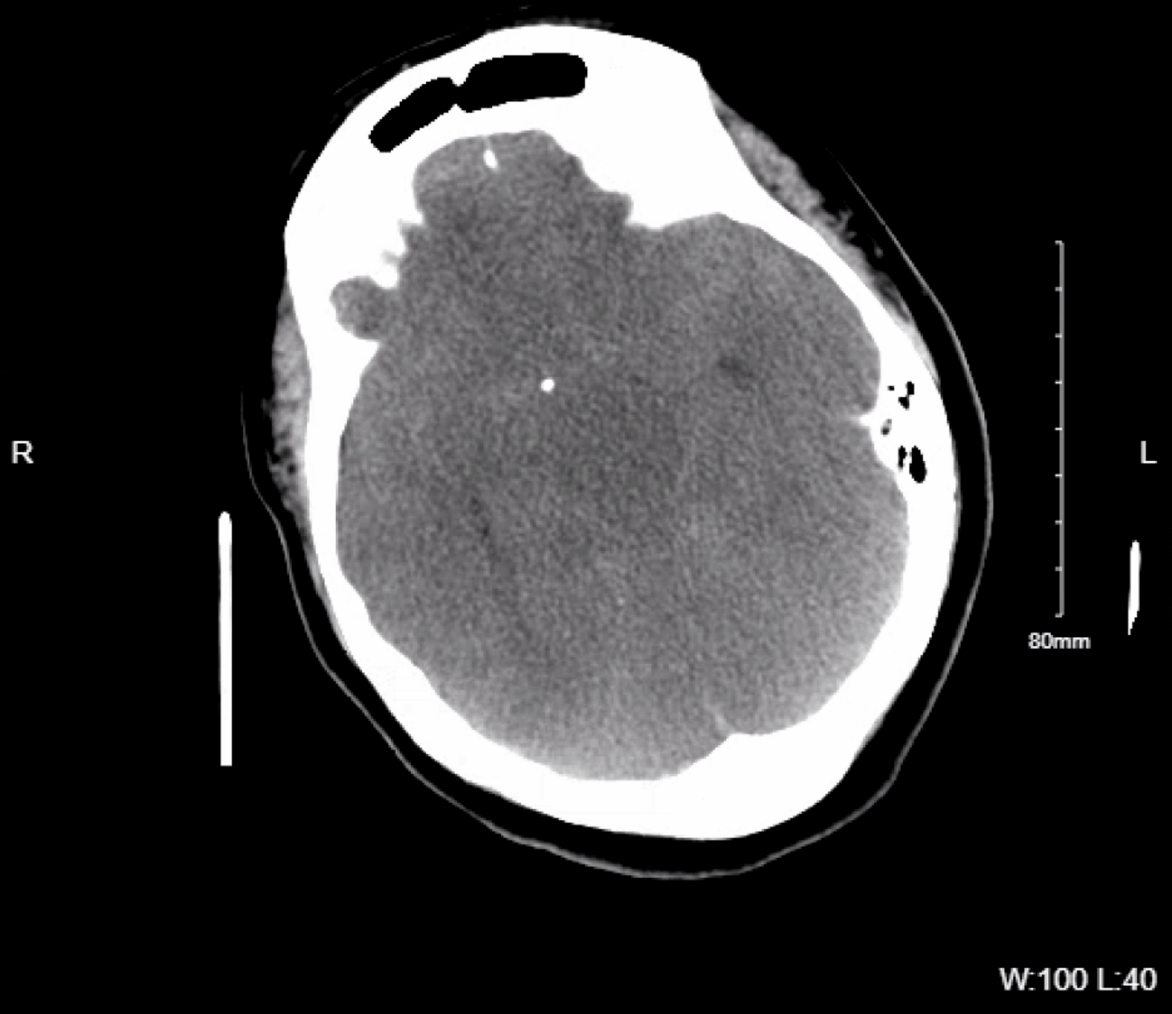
CTH demonstrating diffuse cerebral edema with effacement of basal cisterns and early temporal horns indicative of elevated intracranial pressure CTH, computed tomography of the head
